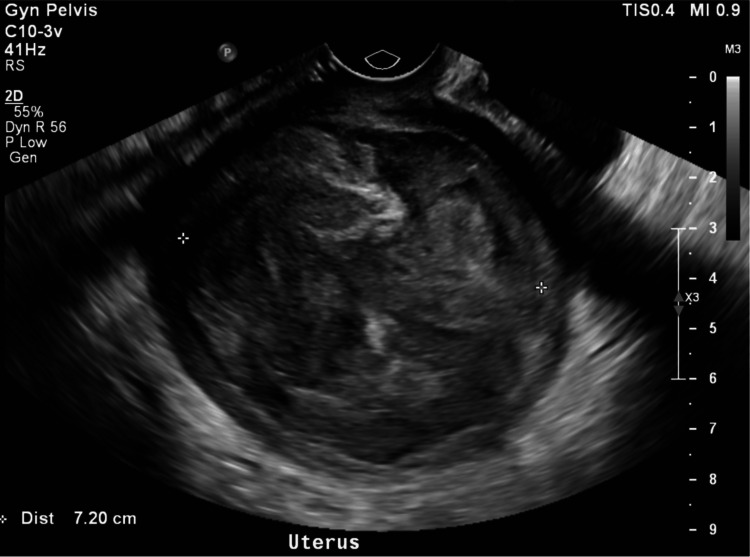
Transvaginal ultrasound of uterine mass A transvaginal ultrasound performed in office showed a well-defined, solid, concentric, hypoechoic mass in the uterus measuring 7.20 cm. The uterus is difficult to visualize due to the size and compression of the mass.
